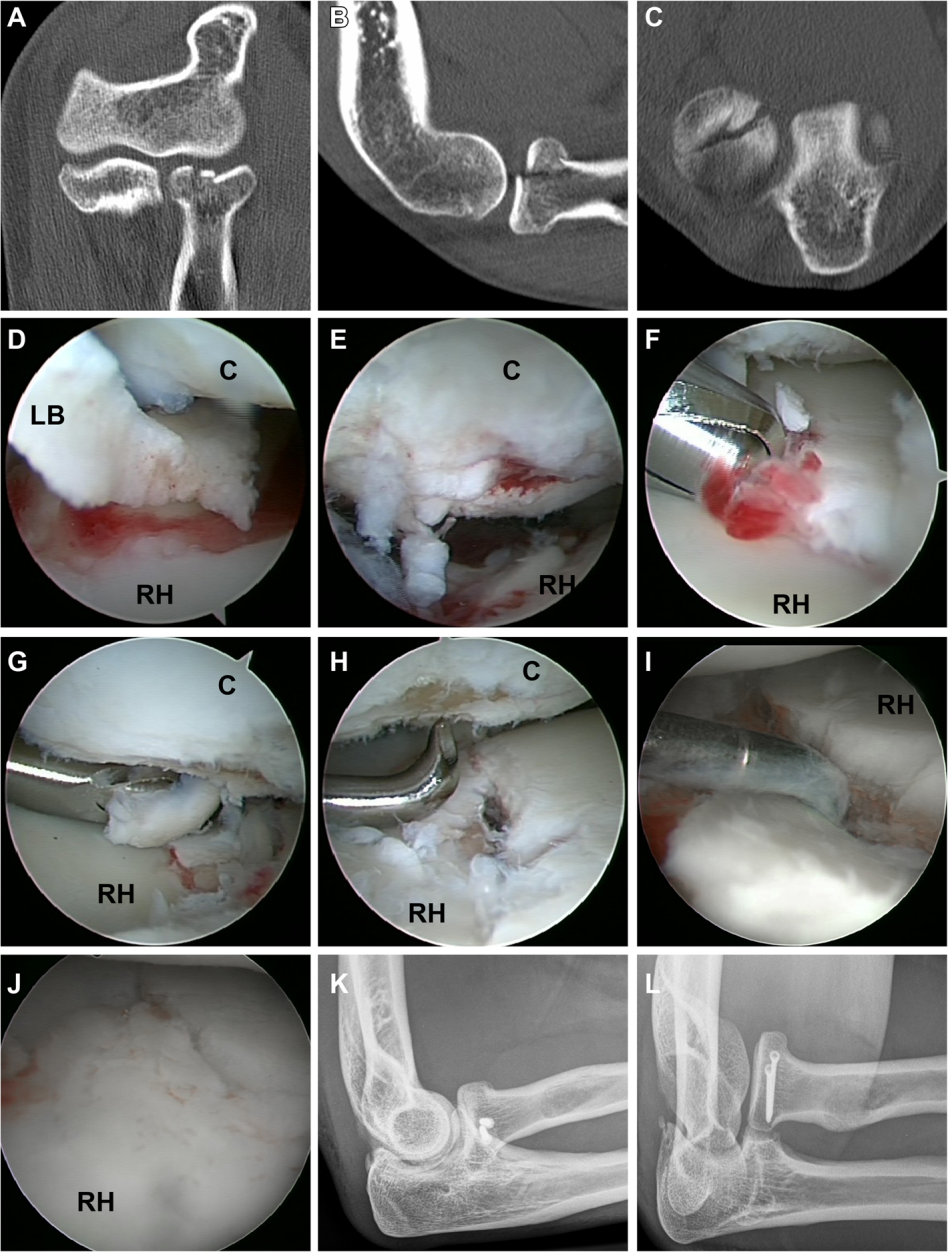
Mason Type II radial head fracture with traumatic capitellar chondral lesion und loose joint bodies. a-c CT scan showed a dislocation of 3 mm in coronal view. Loose bodies and capitellar injury were not identified (d) Arthroscopy revealed a large chondral loose body [LB] entrapped between the capitellum [C] and the radial head [RH]. e grade IV chondral lesion to the capitellum. f After removal of fracture hematoma, (g) chondroplastic and (h) microfracturing was performed at the capitellum humeri. i Fracture reduction was carried out with a sharp hook and (j) anatomic restoration of the radial head was achieved by screw osteosynthesis over the anterolateral portal. k,l Postoperative x-rays demonstrate anatomic reduction and correct screw placement
